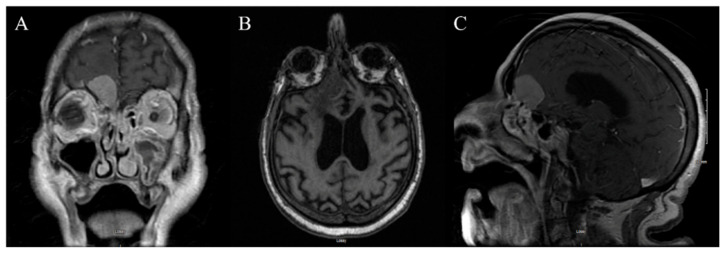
Case 2’s preoperative contrast-enhanced MRI of a dura-attached enhanced 3.2 × 2.4 cm lesion in the anterior cranial fossa suggestive of a meningioma. (A) Coronal view shows compression of the basal surface of the frontal lobe. (B) Axial view shows blunted anterior horn of the right lateral ventricle. (C) Sagittal view shows compression of the basal surface of the frontal lobe.
